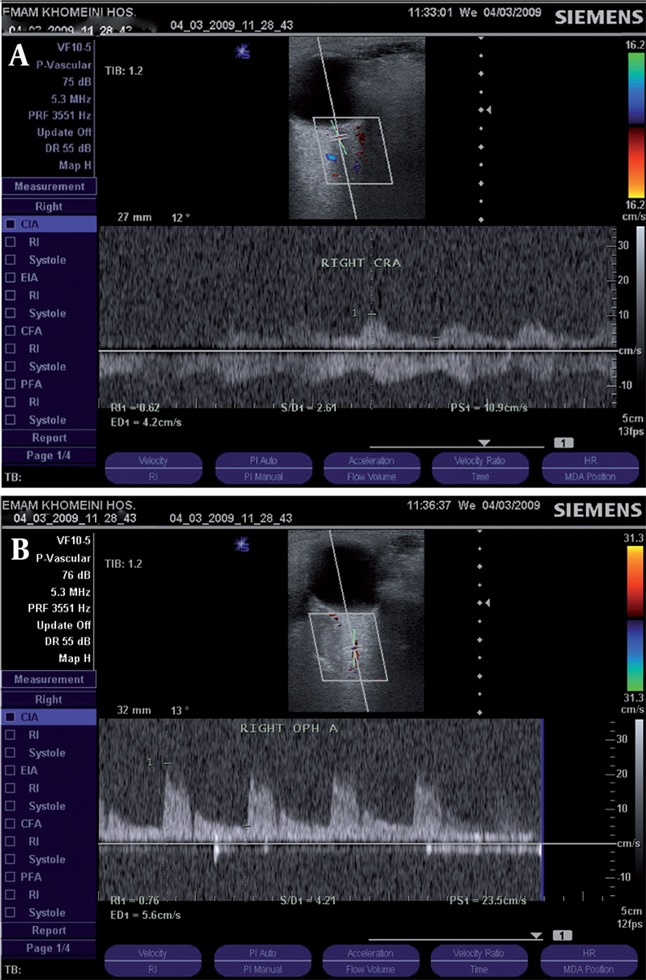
A 23-year-old female with PCKD and a history of 5-month dialysis. A, Doppler spectrum of the central retinal artery (CRA); B, Ophthalmic artery (OA)
